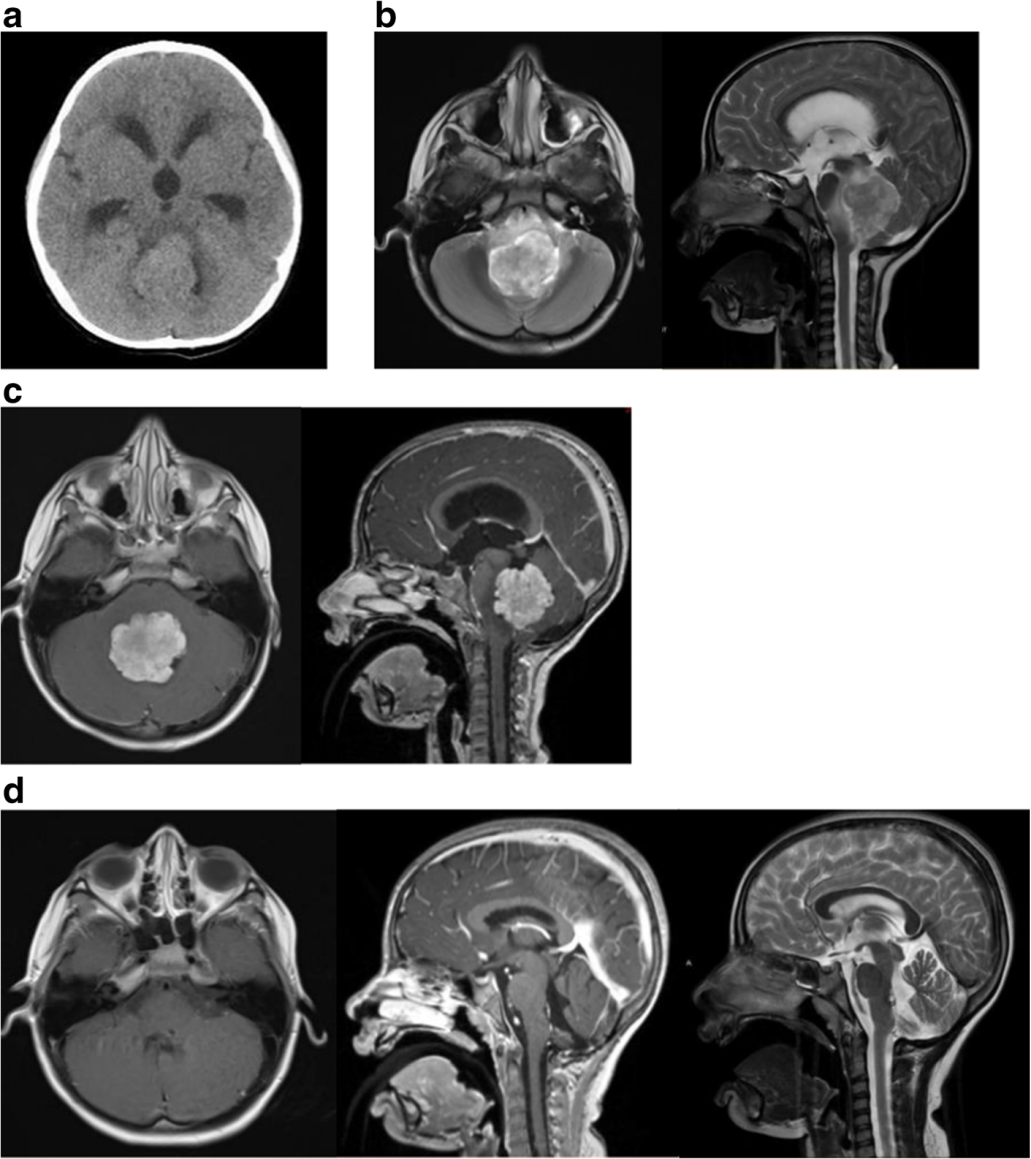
Case 7. a Non-contrast enhanced CT showing an isodense lesion centered in the fourth ventricle with moderate ventriculomegaly. b T2-weighted MR, axial image (left) and sagittal image (right) showing a severe compression on the left-sided floor of the IV ventricle and severe hyperintense changes in the dorsal brainstem and cerebellar peduncle by the IV ventricle tumor. c Contrast enhanced MRI, T1-weighted axial image (left) and sagittal image (right) showing a lobulated homogenously enhancing tumor centered in the IV ventricle. d Postoperative MRI brain; contrast enhanced T1-weighted axial image (left) and sagittal image (center) showing a resolution of IV ventricle tumor with a small residual enhancement on the floor of the IV ventricle, and resolution of brainstem edema on T2-weighted sagittal image (right)
